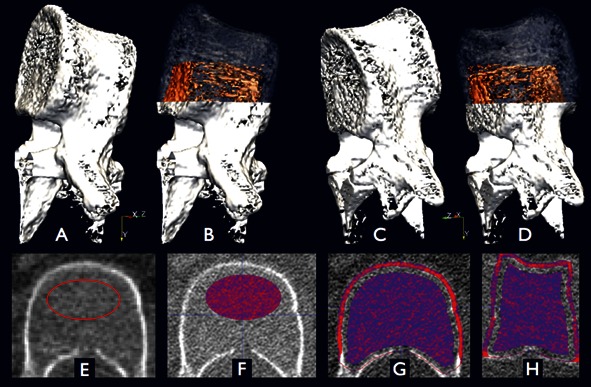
2D and 3D visualization of a vertebra imaged noninvasively by high-resolution quantitative computed tomography. The 3D images of the top row show a vertebra viewed from two angles differing by 180 degrees (*A*, *B* versus *C*, *D*). The semitransparent versions (*B*, *D*) depict the cortical endplates and elements of the trabecular microstructure of a selected subvolume (colored brown within the semitransparent vertebral body). The bottom row shows placement of the volume of interest (VOI) within the vertebral body in the axial (*E*–*G*) and sagittal (*H*) planes. For HRQCT (*F*–*H*) a cortical region (endplates and lateral and anterior cortices depicted on *G* and *H*) and two different trabecular regions (elliptical in *F* and entire volume in *G* and *H*), except a 2-mm subcortical endosteal region, were evaluated. The elliptical region in *F* was defined similar to the standard QCT region shown, for comparison, on a regular resolution QCT image in *E*. Compared to QCT, HRQCT demonstrates improved delineation of cortical bone (*E* versus *F*).
